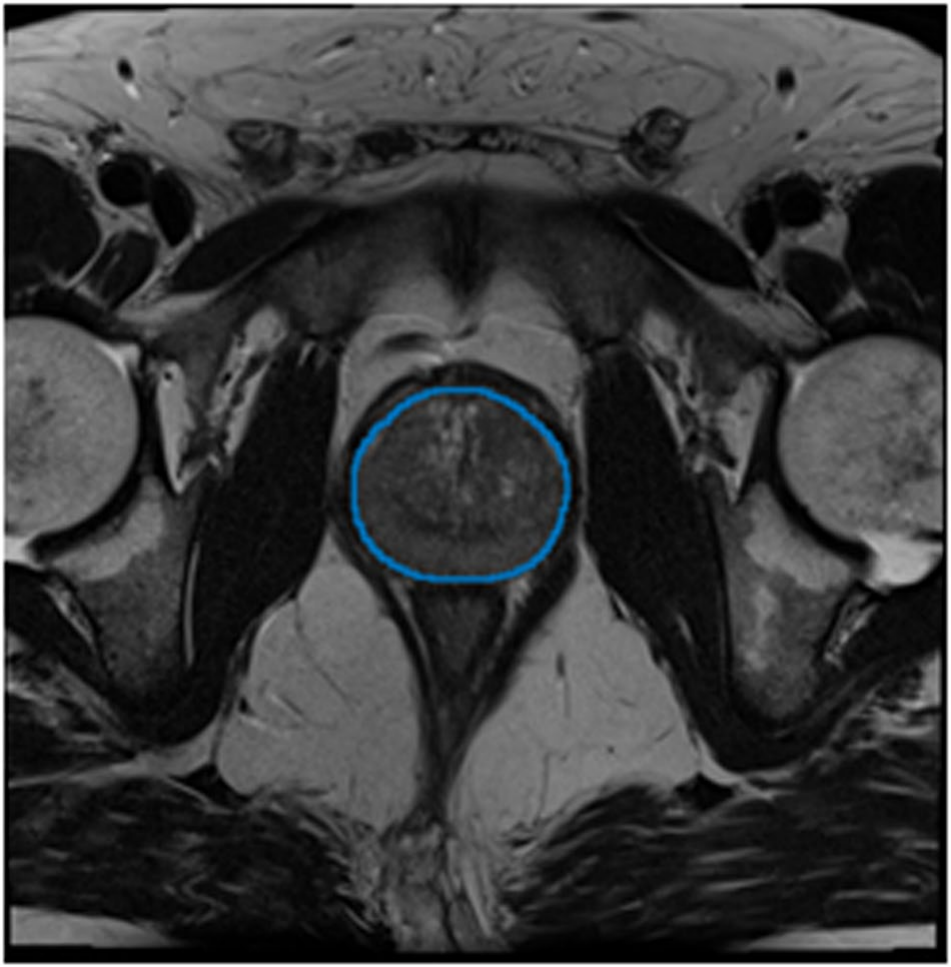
Automatic segmentation of prostate gland from T2 MRI scan of patient ProstateX-0336.
